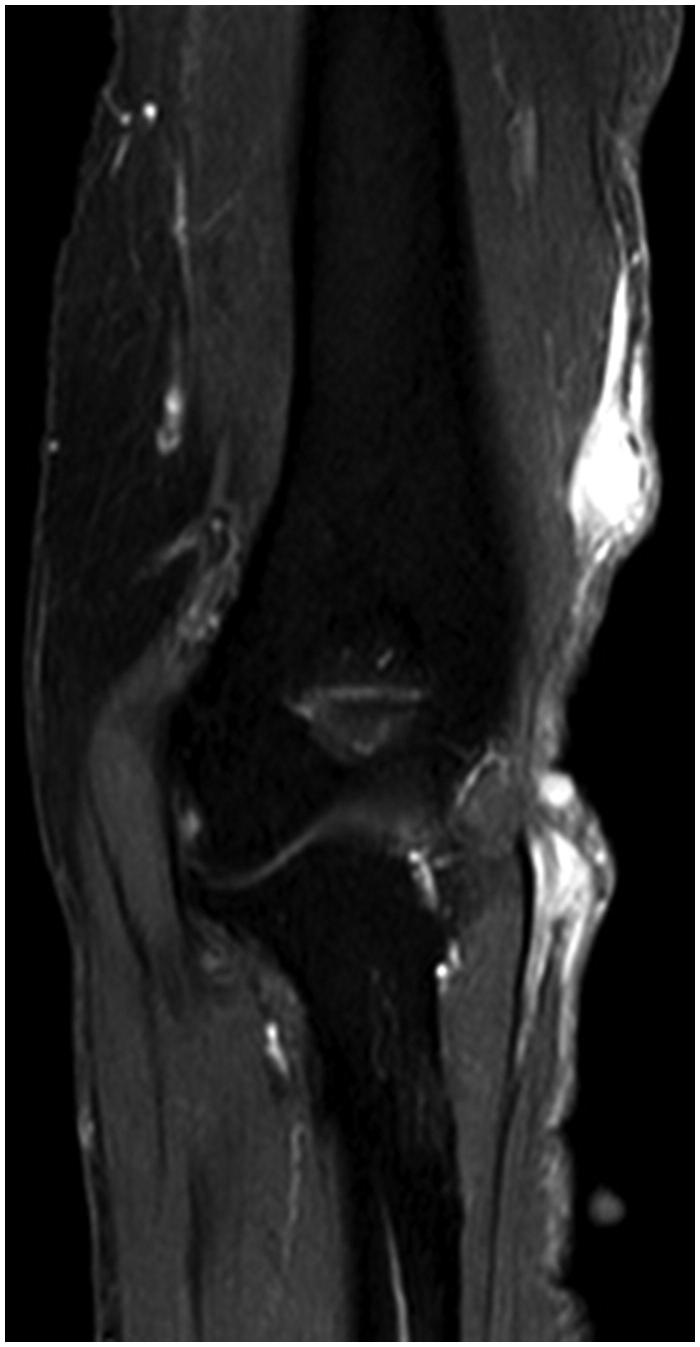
Pre-operative magnetic resonance image, showing the coronal Gd-DTPA-enhanced T1WI.
